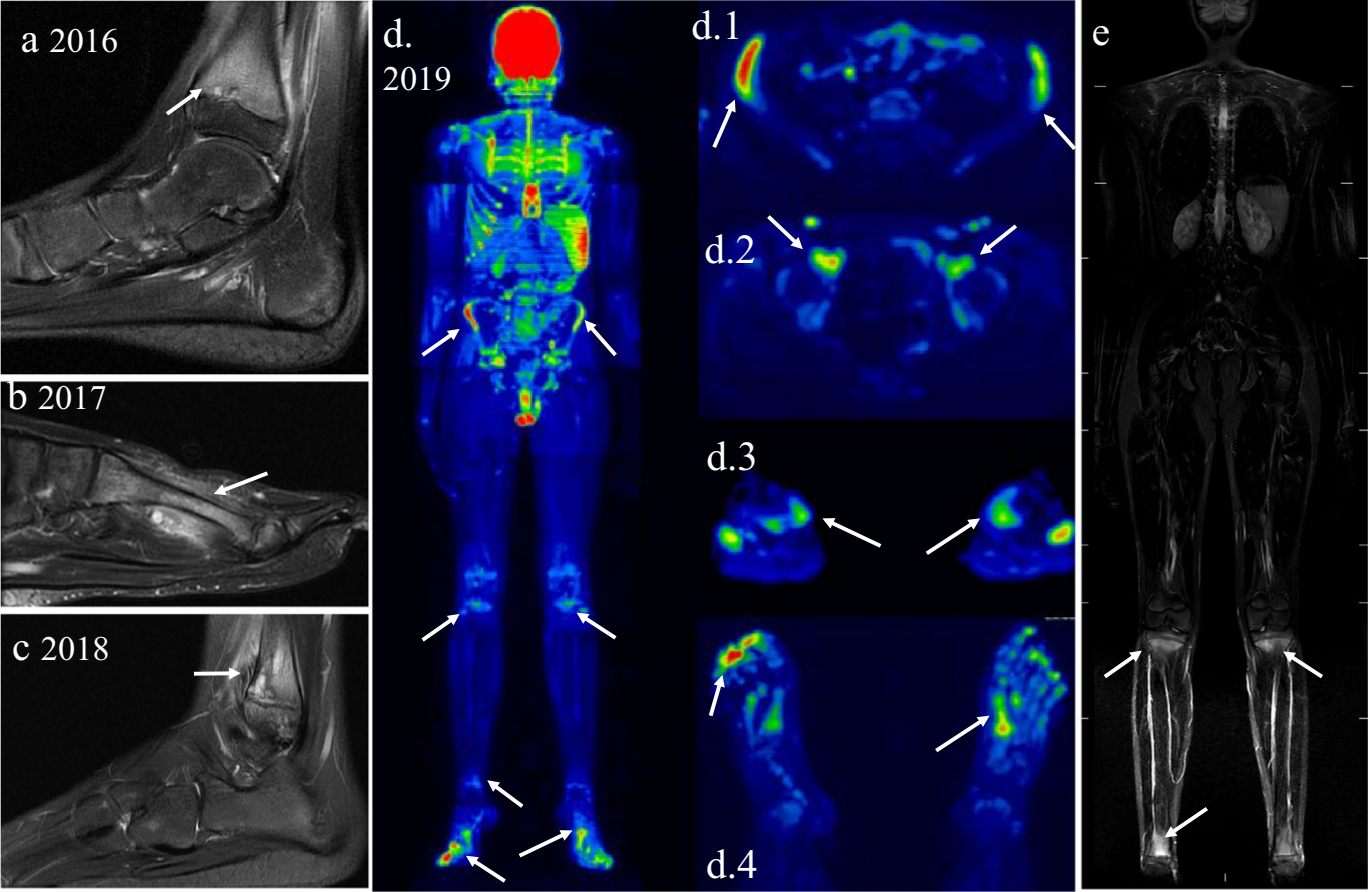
A 10-year-old boy who complained of pain in his left ankle and presented with a metaphyseal lesion in the distal tibia (a) which was initially suspected of bacterial osteomyelitis, despite negative biopsy and culture. In 2017, a foot examination showed a lesion in the second metatarsal of the left foot (b) and in the following year, lesions in the distal fibula were detected (c), which led to the hypothesis of CNO. WB-MRI was then performed and showed multiple lesions dispersed throughout the skeleton, in typical bilateral locations, confirming the diagnosis of CNO. WB-MRI DWI (d) and STIR (e) detected involvement of the iliac bones (d.1), anterior acetabulum column (d.2), bilateral tibial proximal metaphysis (d.3) and bones of the metatarsus (d.4) in correspondence with the STIR sequence of the whole body (e)
